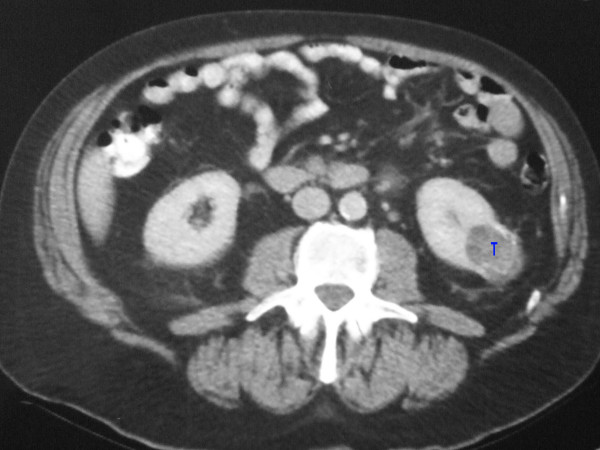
CT picture of a left lower pole renal tumour (T).
